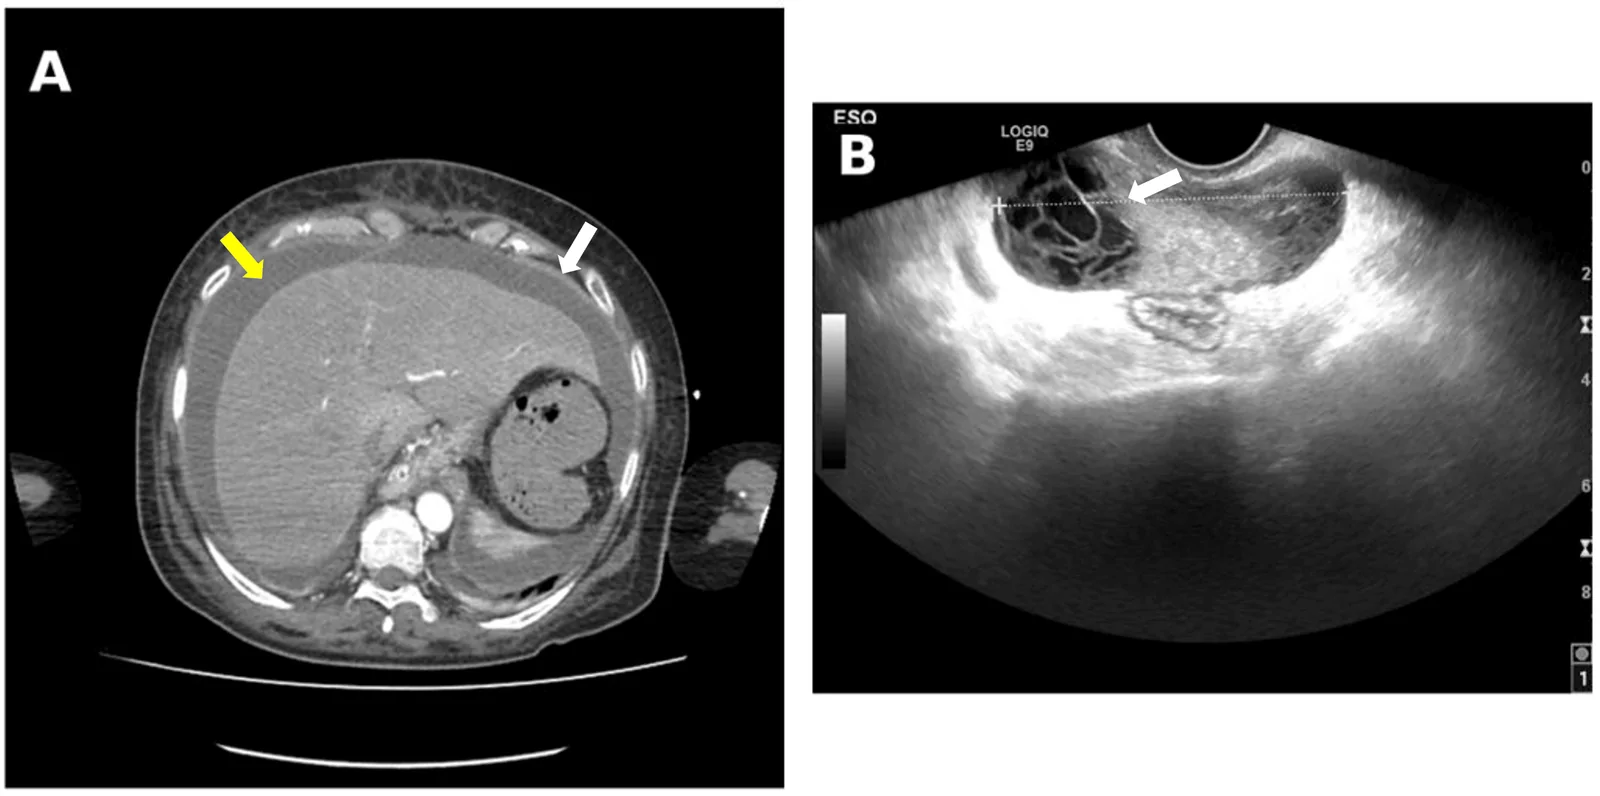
Imaging findings initially suggesting malignancy (A) Axial contrast-enhanced computed tomography showing voluminous ascites (yellow arrow) and diffuse peritoneal thickening with enhancement (white arrow), initially raising concern for peritoneal carcinomatosis. (B) Pelvic ultrasound demonstrating a multiseptated, multicystic left ovary (white arrow), interpreted as a possible ovarian neoplasm.
